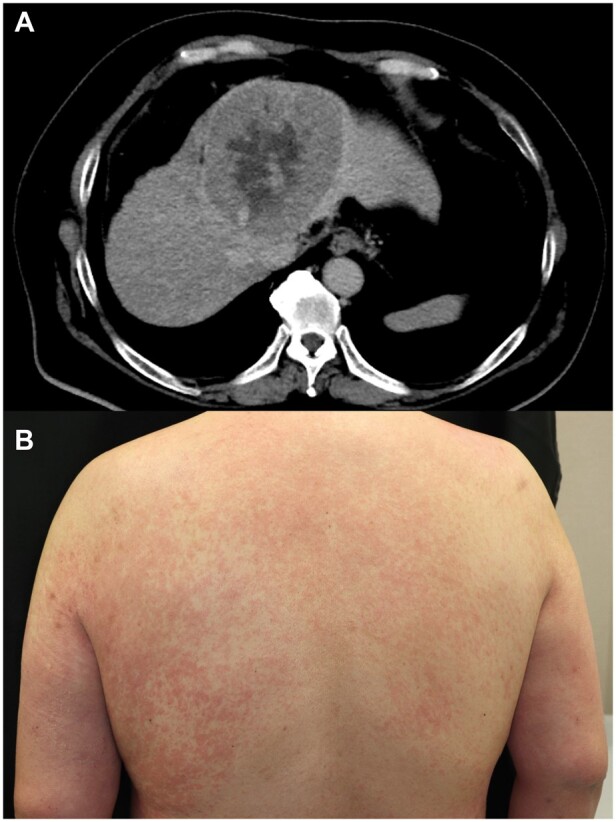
CT image of hepatocellular carcinoma and clinical image of skin lesions. (A) Contrast-enhanced CT revealed a tumour with a long axis of 10 cm in the S4 segment of the liver. Necrotic changes were visible in the central region. (B) Extensive coalescing oedematous erythema was observed on the limbs and trunk
